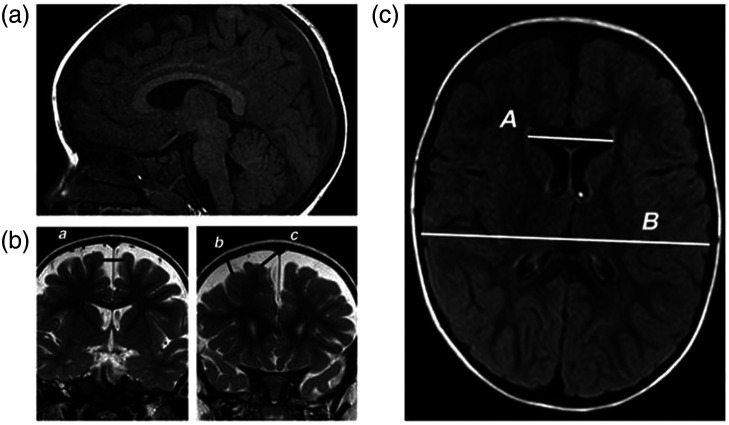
Example of a 33-month-old female born in a eutocic delivery (gestational age: 35 weeks, weight = 2020 g, height 45 cm, and HC = 33 cm), with an Apgar score (that is a standard neonatal health assessment score) of 6-9-9 at 1, 5, and 10 min of delivery. She was referred to a pediatric neurosurgeon for evaluation of hypotonia and enlargement of subarachnoid spaces. MRI showed the characteristic findings of benign enlargement of subarachnoid spaces in the frontal lobes [(a) sagittal T1-weighted MRI and (b) coronal T2-weighted MRI images): a, size of the interhemispheric fissure (12 mm); b, craniocortical width (8.4 mm); and c, sinocortical width (12.4 mm). (c) (axial T1-weighted MRI), the Evans’ Index (0.29) was calculated as the ratio between the maximum width of the frontal horns of the lateral ventricles (A=31  mm) and the maximum transverse inner diameter of the skull at the same axial slice (B=105  mm).
